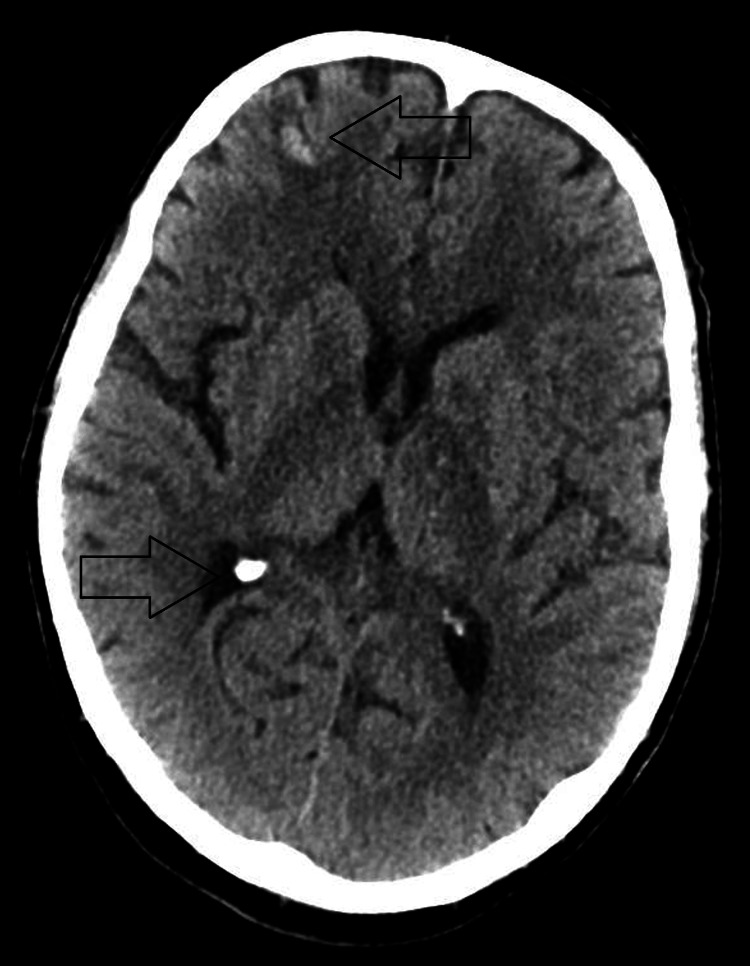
CT head showing hyperdensity lesions suspicious for microhaemorrhages involving the right frontal subcortical grey matter and basal ganglia.
